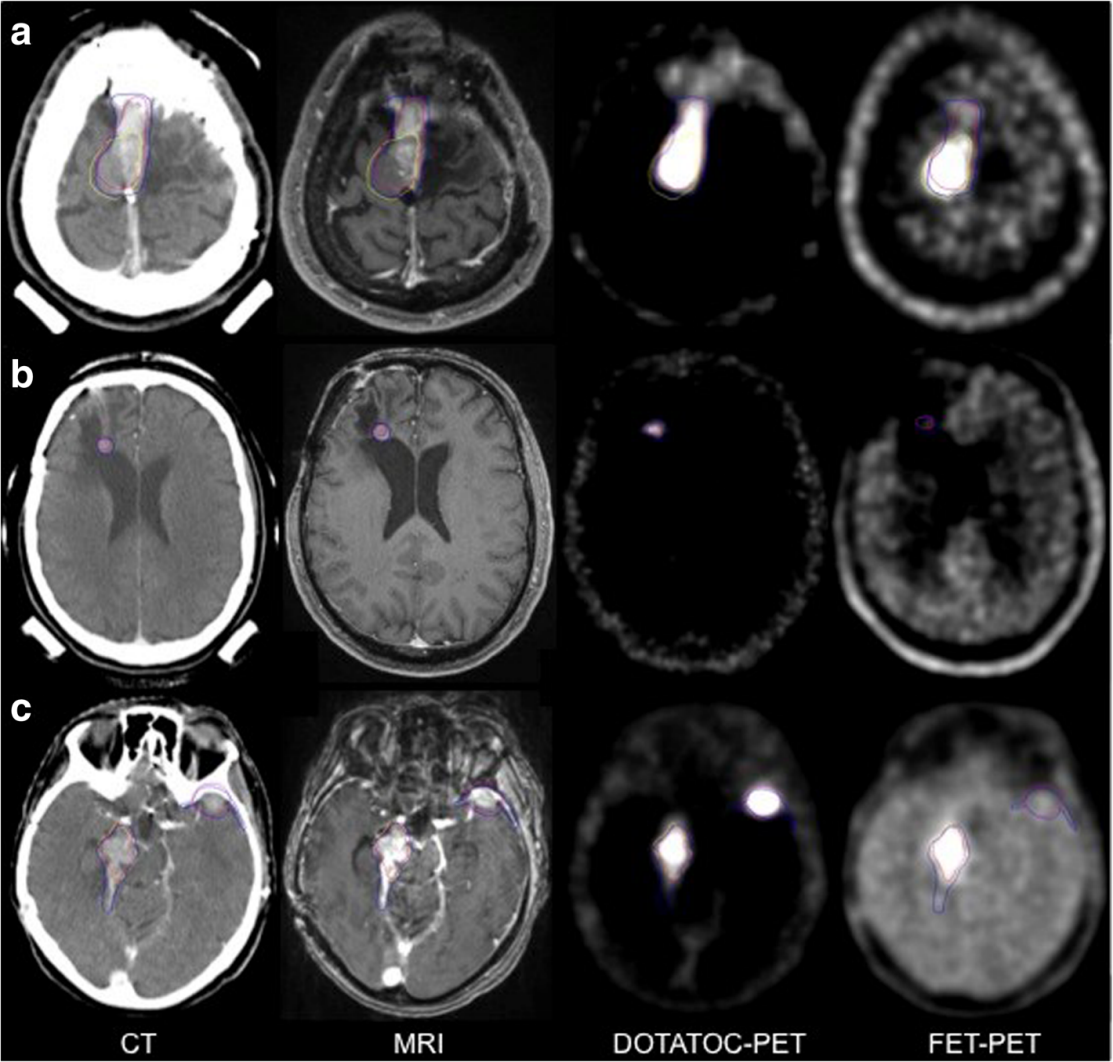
Delineated GTVs of three patients based on CT and MRI (blue), DOTATOC-PET (purple) or FET-PET (yellow): Meningioma is partially FET-negative (a), Meningioma is completely FET-negative (b). Patient C (c) is suffering from two meningiomas, one FET-positive and the other FET-negative. All meningiomas are clearly visible in DOTATOC-PET, CT and MRI
